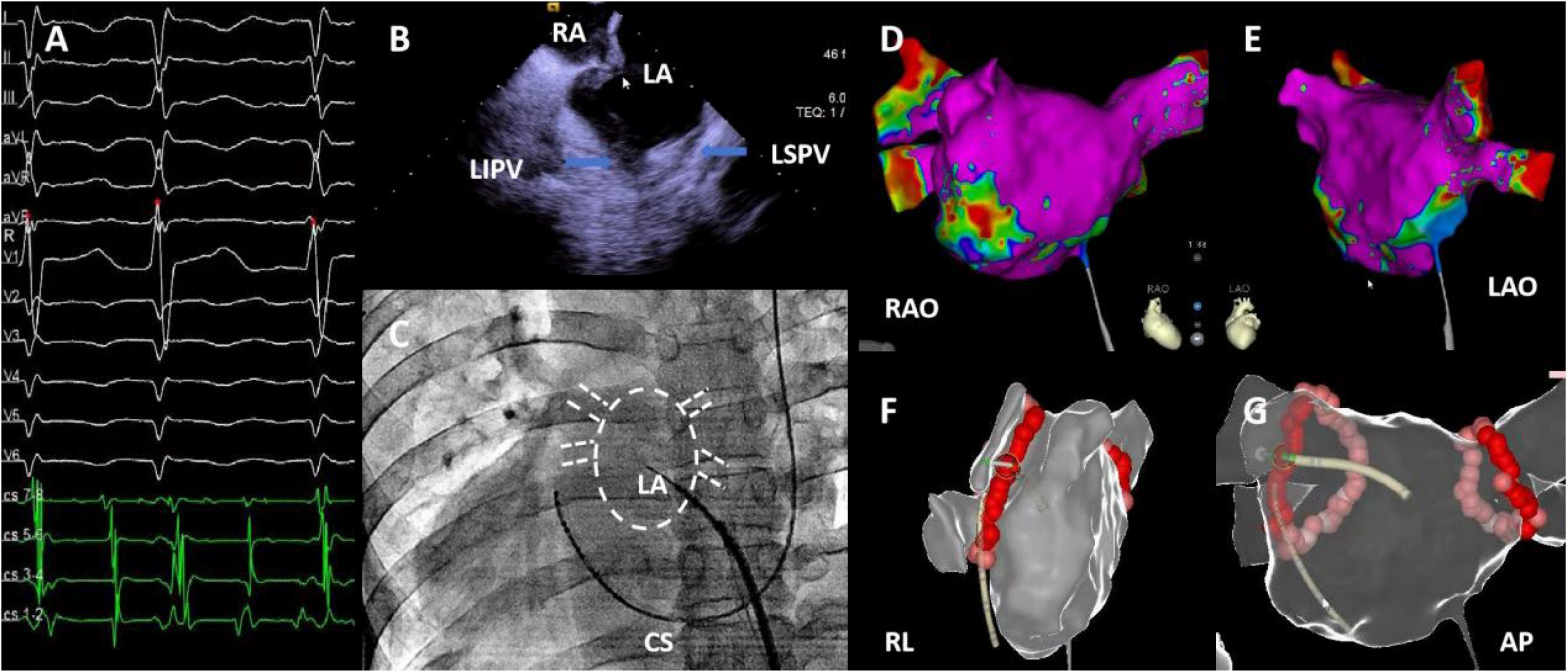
A: Electrophysiology study shows atrial stimulation inducing arrhythmia; surface and intracardiac electrograms indicate atrial fibrillation. (B) Intracardiac echocardiography image showing the puncture sheath (white arrow) aligned with the atrial septum, positioned centrally (LA, left atrium; RA, right atrium; LSPV, left superior pulmonary vein; LIPV, left inferior pulmonary vein). (C) X‐ray in the anterior–posterior view showing the transseptal puncture needle passing through the fossa ovalis into the left atrium (CS: coronary sinus electrode). (D) Right anterior oblique (RAO) 30° three‐dimensional reconstruction model with substrate mapping (purple indicates healthy atrial substrate). (E) Left anterior oblique (LAO) 45° three‐dimensional reconstruction model with substrate mapping. (F) Right lateral (RL) three‐dimensional reconstruction model showing circumferential pulmonary vein ablation points (red dots indicate ablation sites). (G) Anterior–posterior (AP) three‐dimensional reconstruction model showing circumferential pulmonary vein ablation points (red dots indicate ablation sites).
